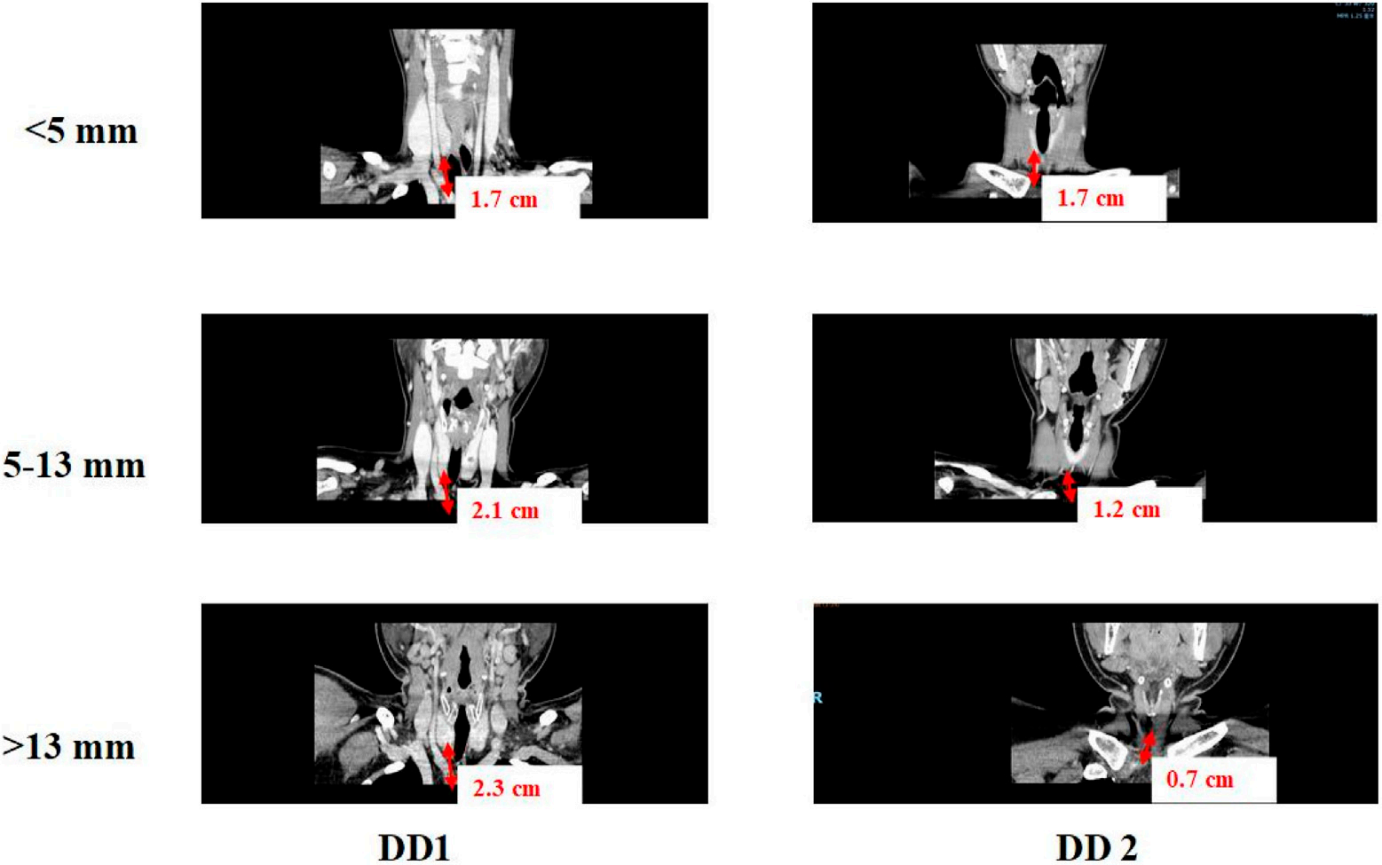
CT images to determine the values of DD1 and DD2.
